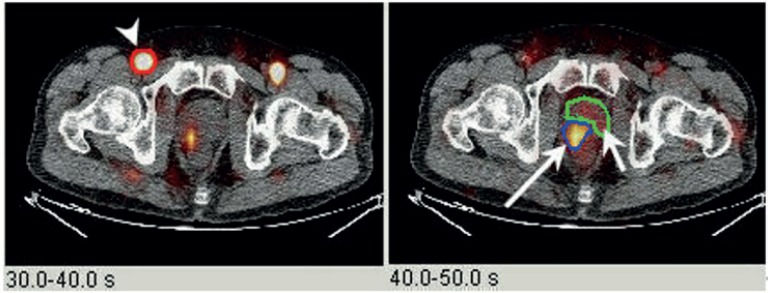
^11^C-choline PET / CT dynamic blood flow. Axial fused PET / CT sequential blood flow images (left: 30-40 seconds after injection; right: 40-50 seconds after injection) showing preferential arterial supply to the prostate tumor relative to background prostate tissue (arrowhead = iliac artery; large arrow = prostate cancer; small arrow = background prostate tissue).
